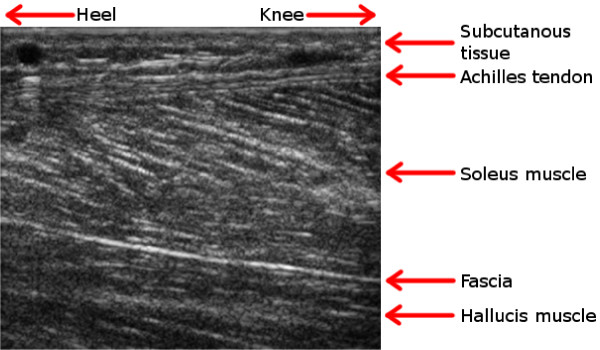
An ultrasound image. The anatomic regions in the area around the Achilles tendon are explained by this example ultrasound image. All ultrasound images that occur in this article are captured at about the same anatomic region and are similar to this image. Case Example 1 and Case Example 2 are using a subset of such an image, covering only the Achilles tendon. Case Example 3 exploits the full image.
